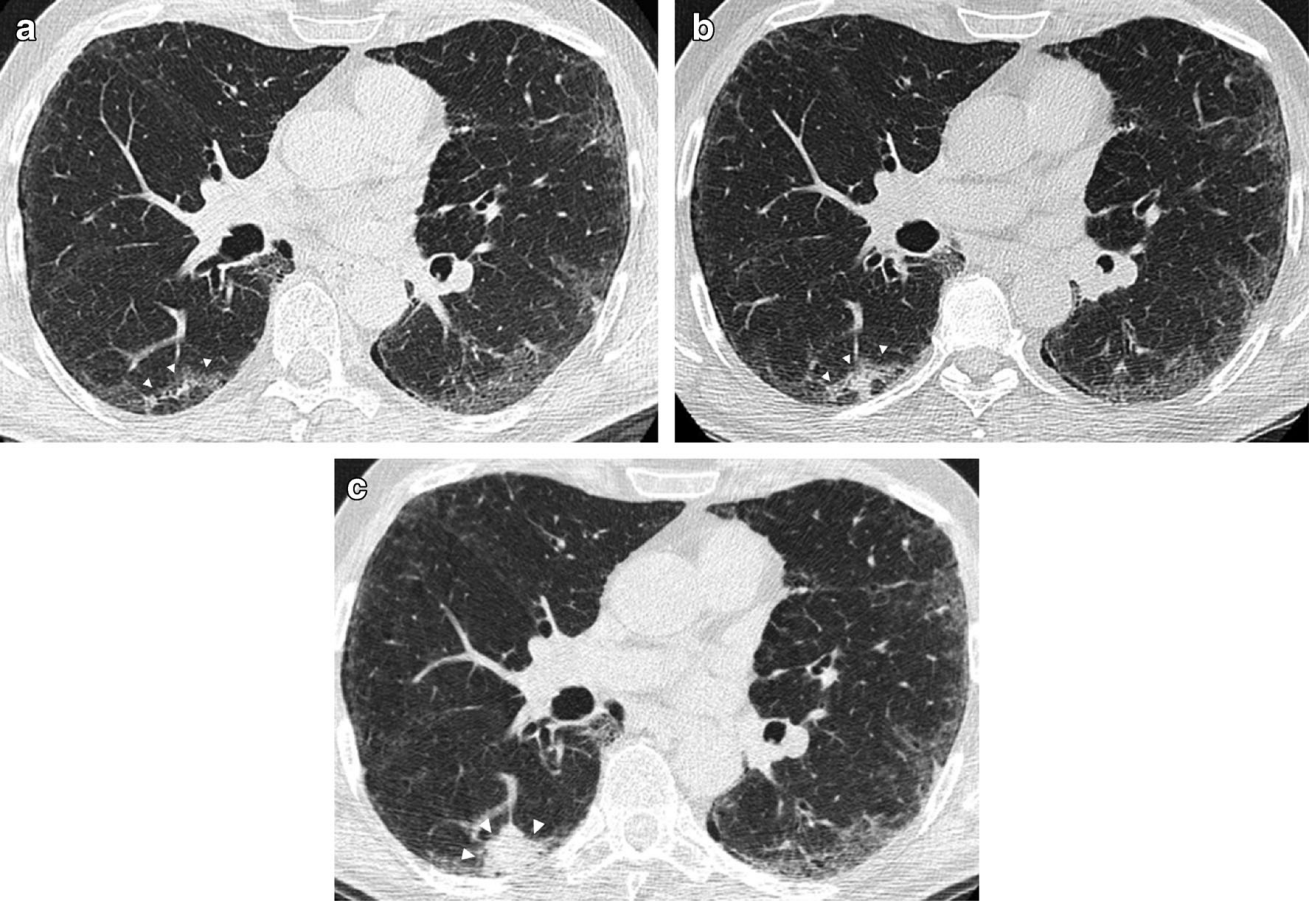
Lung cancer arising within a pre-existing fibrotic lung disease. (a) Low-dose CT demonstrates a bilateral subpleural reticular pattern and a ground-glass opacity, with a subpleural curvilinear opacity in the right lower lobe (*arrowheads*). (b) Low-dose CT scan obtained 1 year later confirmed the fibrotic disease with an increased density of the subpleural opacity in the right lower lobe (*arrowheads*), interpreted as a progression of the fibrosing pattern. (c) At follow-up CT performed 1 year later, there is a remarkable increase of subpleural opacity in the right lobe with the development of a 32-mm mass, resulting in lung adenocarcinoma (*arrow*)
